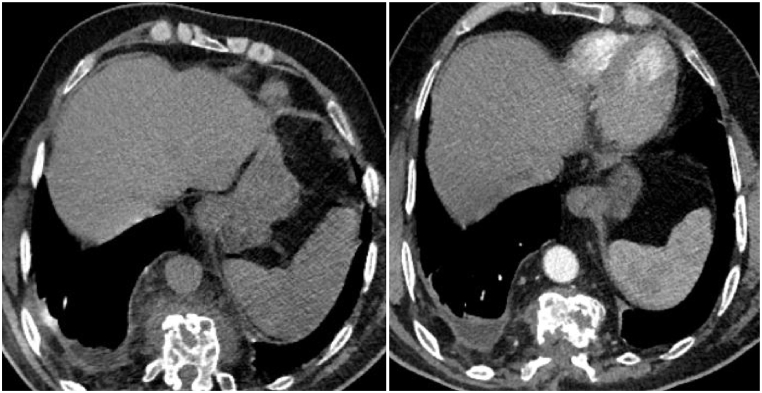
CT scan cross section at T11 vertebra (2020 on the left and 2022 on the right) showing a mild pleural effusion.
